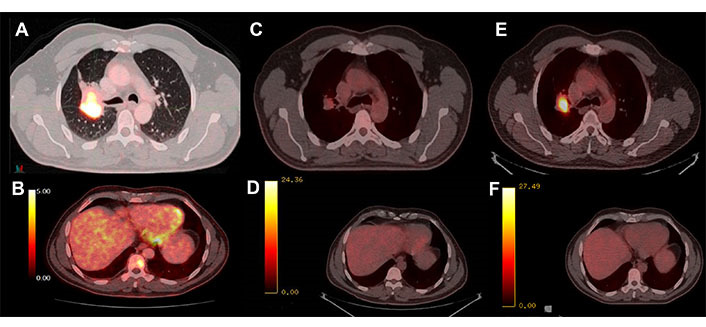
PET-CT with 18-fluorodeoxyglucose (FDG). (A) Hypermetabolism of the basal parahiliar right mass; (B) hypermetabolism of the bone metastasis at D9; (C) initial response to osimertinib in the lung mass; (D) initial response to osimertinib in the bone lesion; (E) progression of the parahiliar mass after 32 cycles of treatment; (F) complete response maintained in the bone
